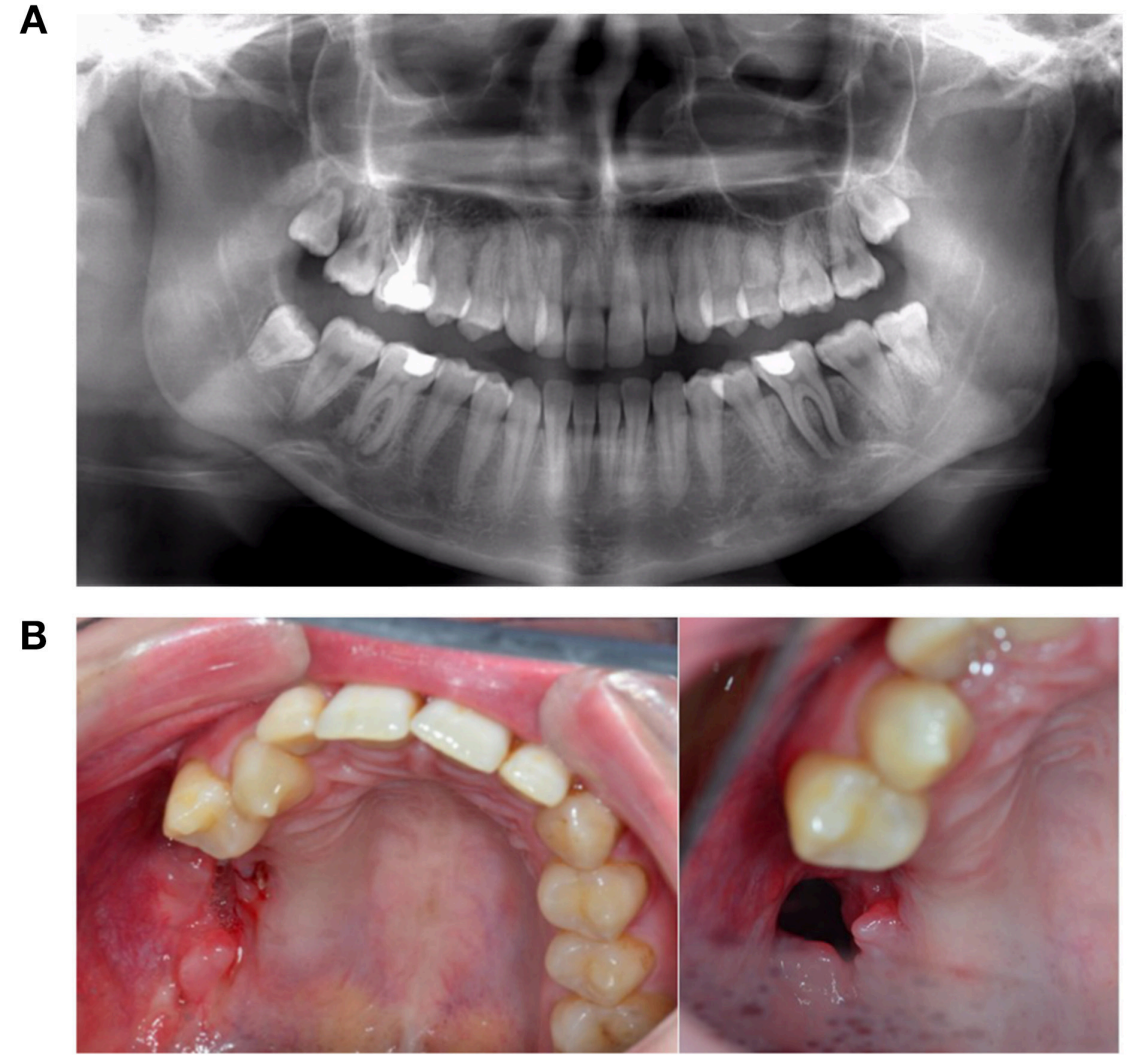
A 25-year-old female with progressive necrosis of the maxilla, showing loss of all molars in the right maxilla and the alveolar bone, with the emergence of a buccosinusal connection that occurred after ustekinumab use for Crohn's disease treatment. (A) A panoramic radiography from the first consult, where the complaint of loose teeth was formulated. (B) Three months after the radiography in panel A, we see the loss of elements 18, 17, 16, and 15. (Photo left). Two months later, an antrum perforation spontaneously occurred.
